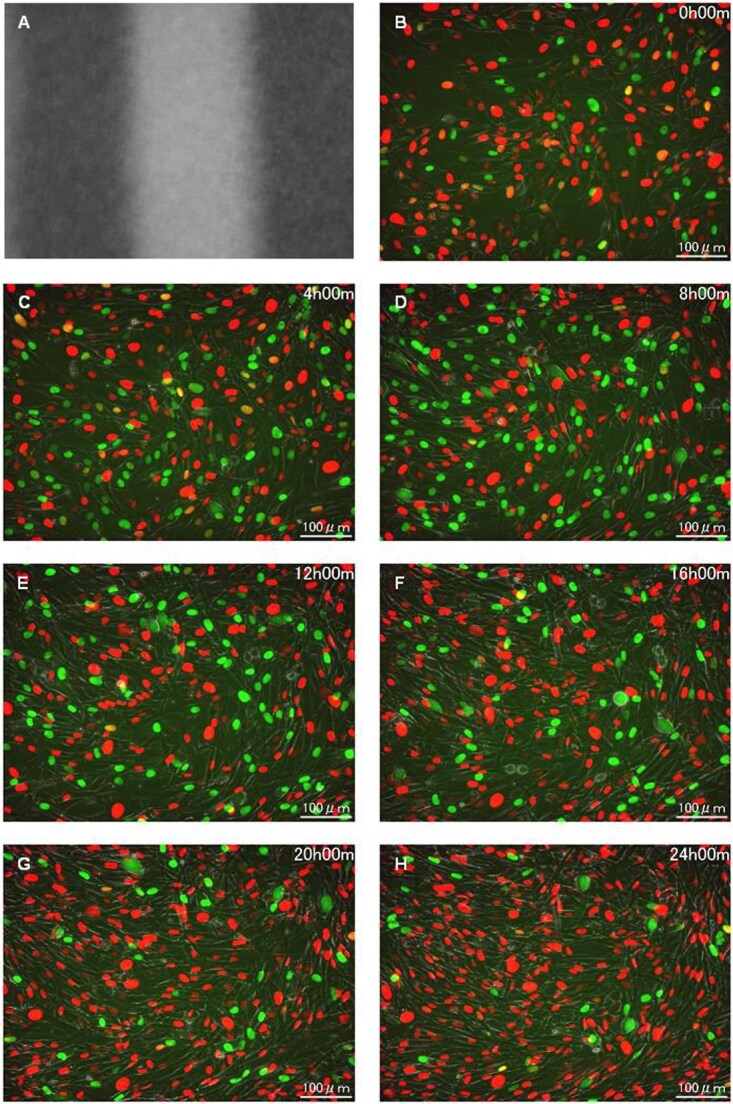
Magnified time-lapse imaging on BJ-1-hTERT-FUCCI cells following exposure to spatially fractionated X-rays. (A) Dose profiles of the 300-μm-slit field using a Gafchromic XR-RV3 radiochromic film. (B–H) Representative magnified fluorescent images of BJ-1-hTERT-FUCCI cells observed 20× magnification objective lens at 0 (B), 4 (C), 8 (D), 12 (E), 16 (F), 20 (G) and 24 h following exposure to the 300-μm-slit X-rays (H). Cell nuclei are indicated by the red, yellow, green and colorless regions depending on their cell-cycle phase, G1, G1/S, S/G2 and M, respectively. Scale bar—100 μm.
